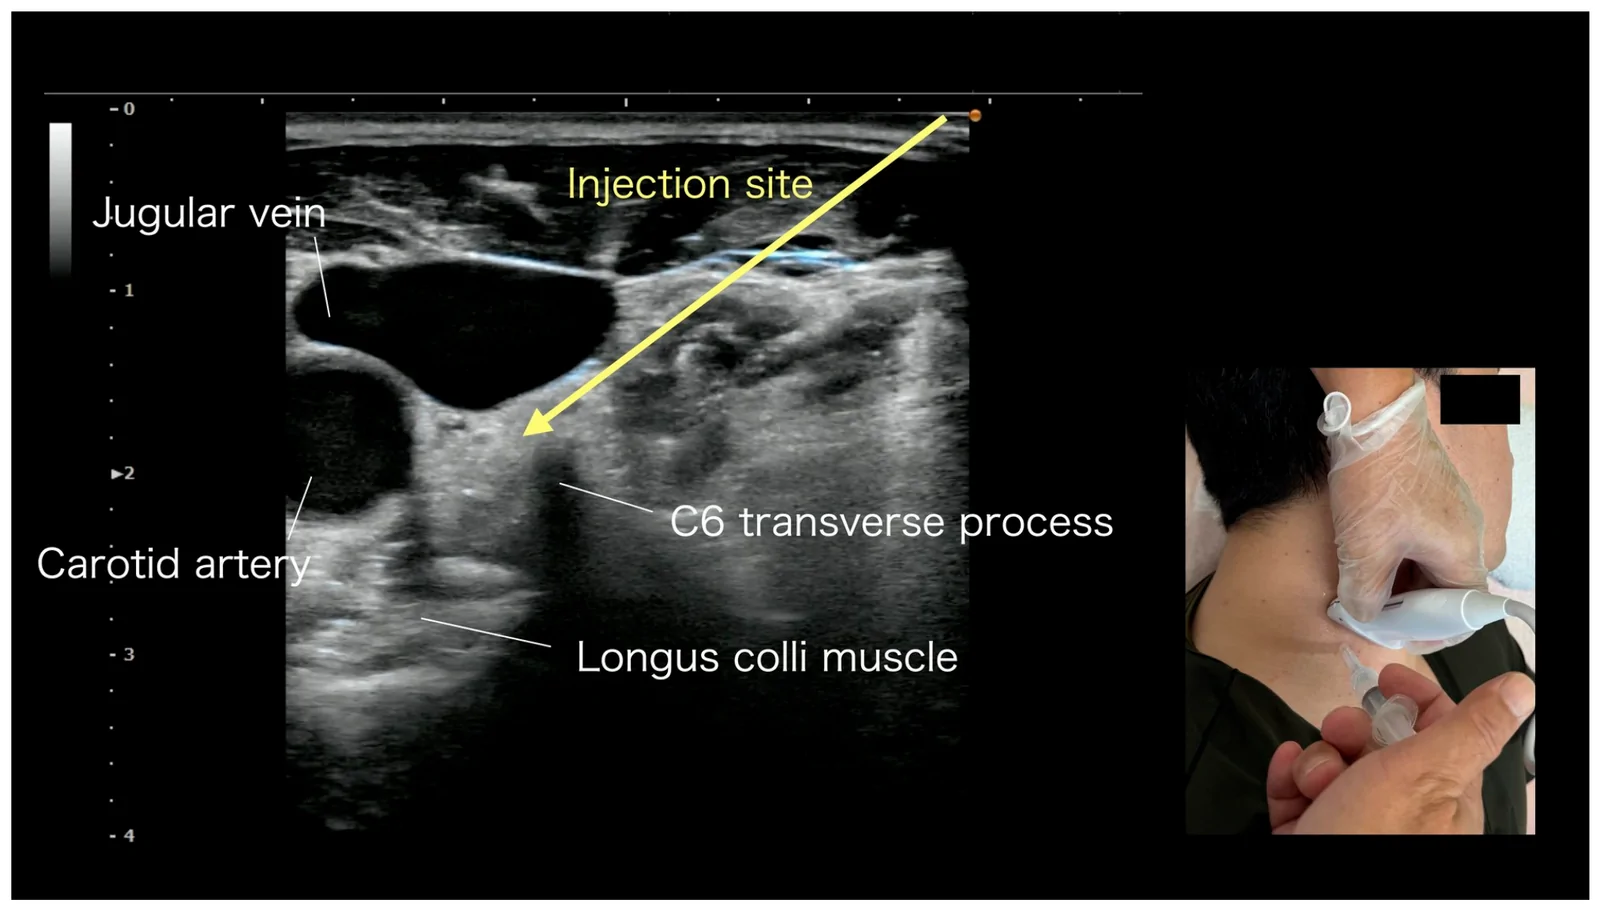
US-FHR around the cervical sympathetic ganglion region (centered on the middle cervical ganglion, extending cephalad and caudad to include the superior cervical and stellate ganglia) (POINT 9). Ultrasound image: Internal jugular vein, common carotid artery, and longus colli muscle are identified. The yellow arrow (injection site) indicates the perivascular fascial space surrounding the cervical sympathetic trunk. Color Doppler identification of vascular structures is mandatory for safety; Doppler is turned off during the release. The inset (bottom right) shows the patient position and probe placement.
